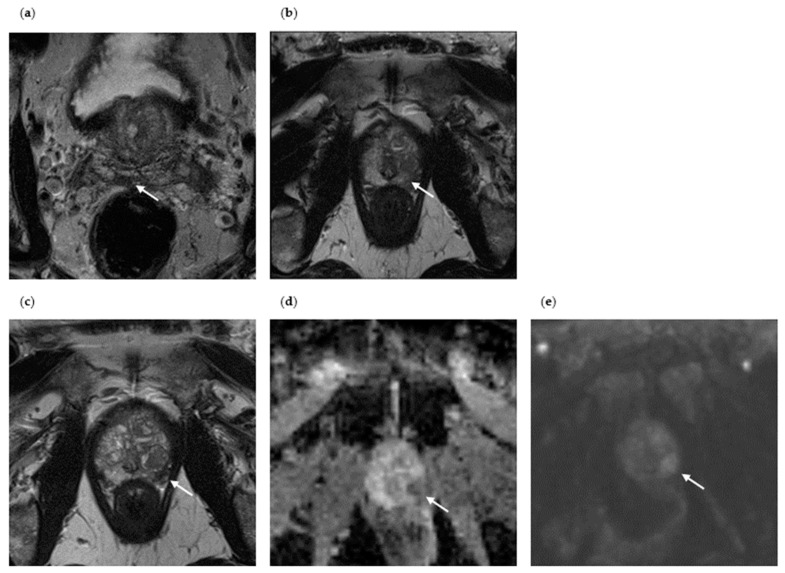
Axial mpMRI of a patient with sPC detection at repeat biopsy (Patient I). (a) Initial T2-weighted mpMRI without PC detection at initial biopsy (PI-RADS lesion 3, the suspicious lesion is marked with an arrow); (b) the PI-RADS 4 lesion visible in the repeat mpMRI does not yet present itself in the initial mpMRI (T2-weighted image); (c) repeat mpMRI of Patient I with sPC detection at repeat biopsy (new PI-RADS lesion 4, T2-weighted); (d) ADC decrease in the PI-RADS lesion 4 at repeat biopsy; (e) signal increase in the high b-value image at repeat biopsy.
